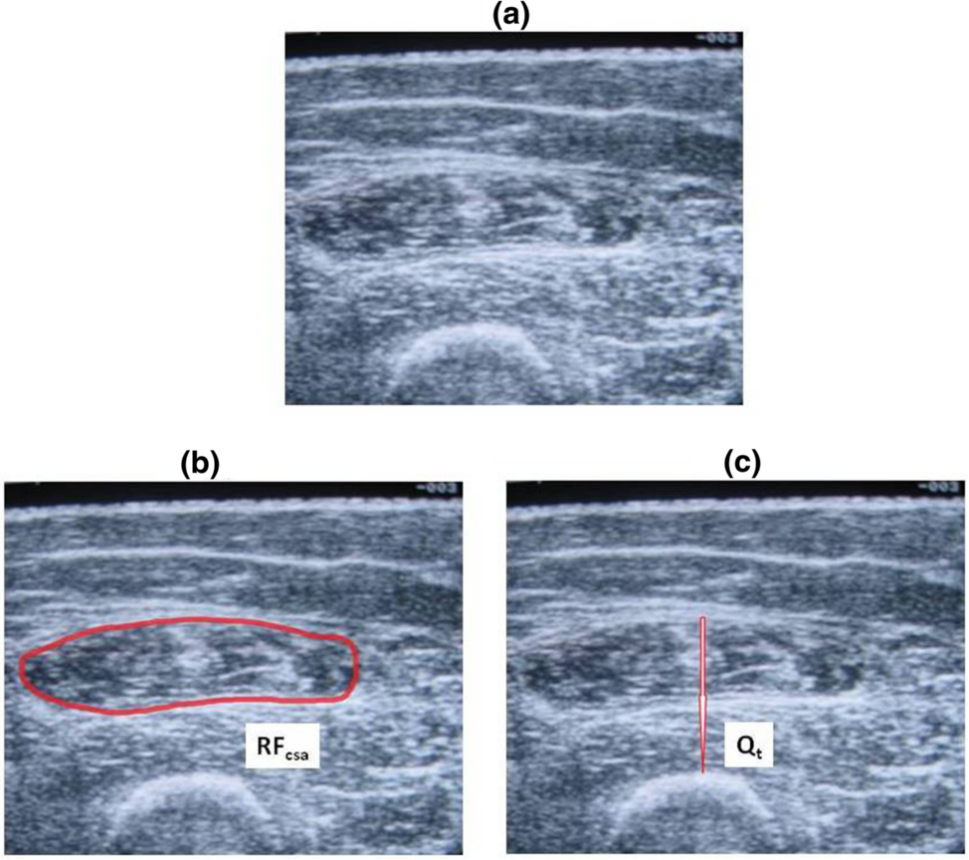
Ultrasound image of the quadriceps. Sample scan of a study participant showing (a) Image at mid-thigh region, (b) RF_csa_, and (c) Q_t_.
